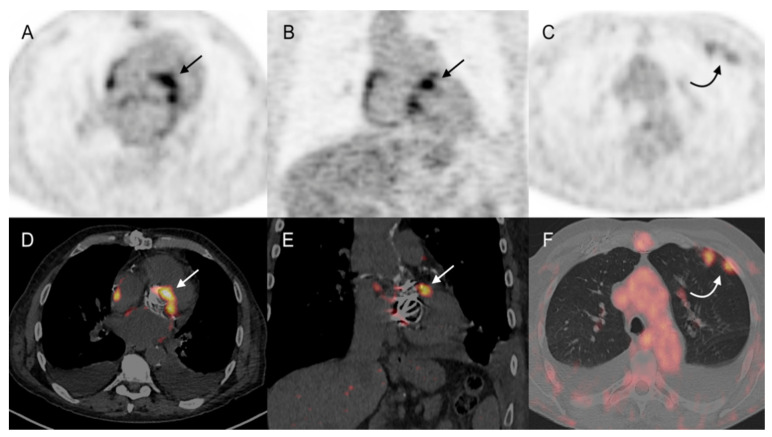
73-year-old man with aortic and mitral mechanic valve prostheses presented with fever and blood cultures positive for Streptococcus gallolyticus (Duke 1/high clinical suspicion degree). TTE and TEE were both negative. PET/CT showed increased focal ^18^F-FDG uptake between the aortic and mitral mechanic valves (arrows) and in left lung parenchymal condensations (curved arrows). According to the Endocarditis Team, final diagnosis was an infected mechanic aortic valve with pulmonary septic emboli. (A–C): attenuation-corrected PET, axial, and coronal slices; (D–F): attenuation-corrected PET/CT, axial, and coronal slices.
